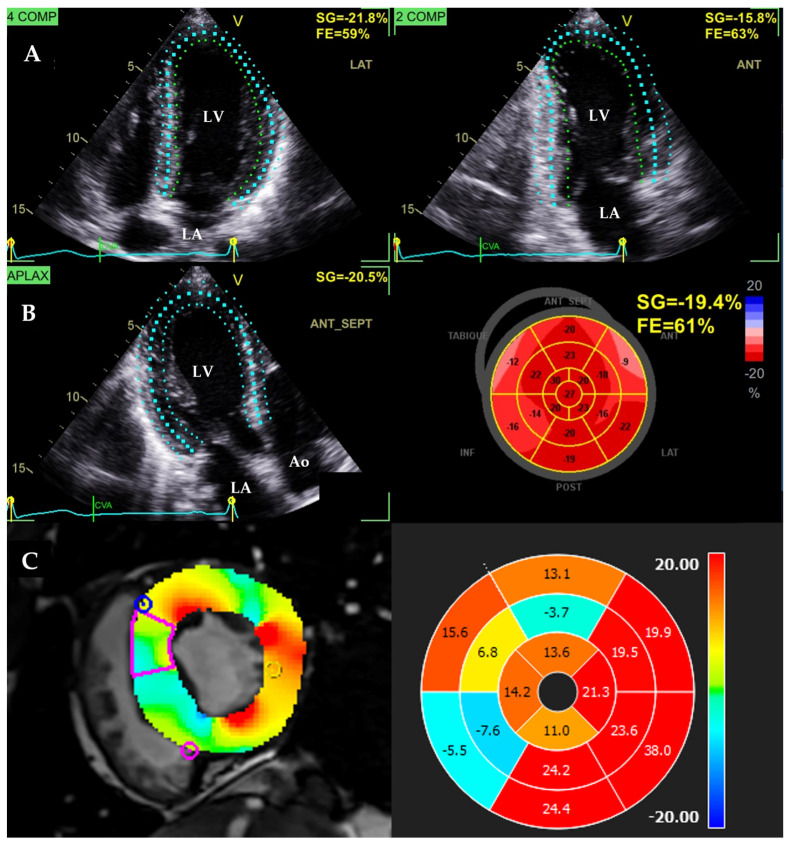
(A,B) LV longitudinal strain (−19.4%) measurement by TTE. (C) LV radial strain measurement using the CMR CVI42 software (Circle Cardiovascular Imaging Inc., Calgary, AB, Canada). Ao: Aorta; CMR: Cardiac magnetic resonance; FE: Fraction ejection; LA: Left atrium; LV: Left ventricle. SG: Strain global; TTE: Transthoracic echocardiography.
